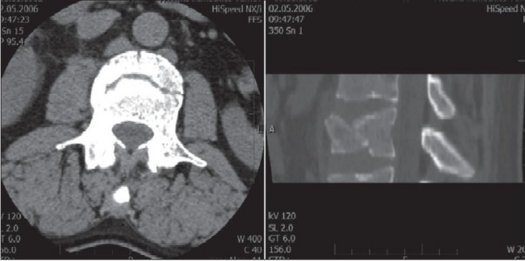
CT scan axial cut and sagittal reconstruction shows a pincer fracture of the vertebral body
